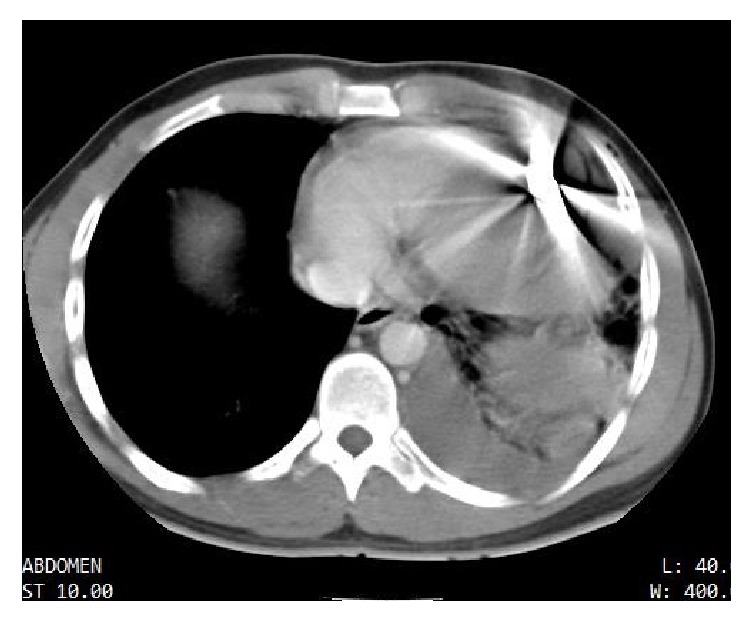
Chest CT at admission shows the bullet near the cardiac apex, not being able to determine if it is inside or outside the pericardial sac.
